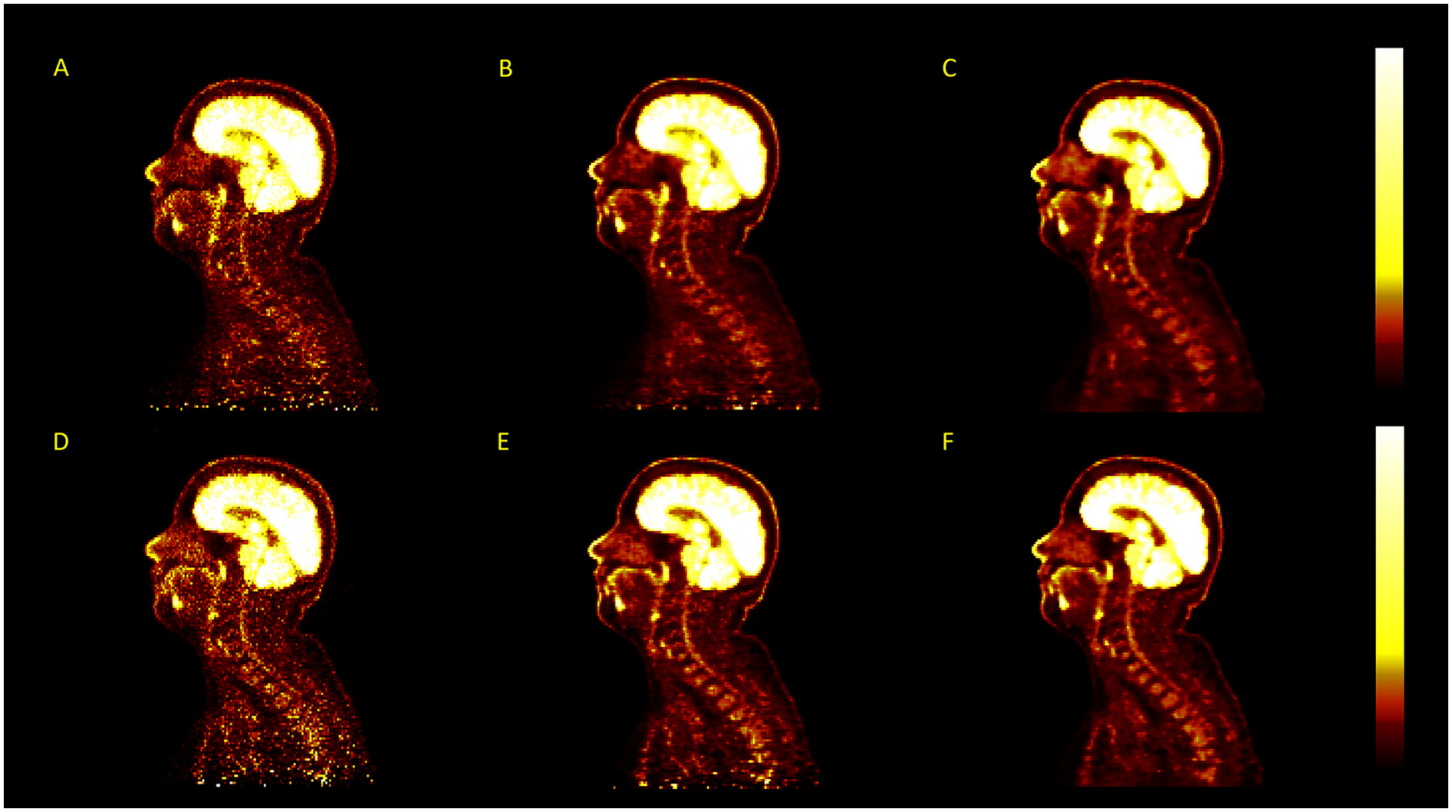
Examples of PET images reconstructed using different image reconstruction algorithms for the same acquisition, with no post-filtering applied, displayed in ${\mathrm{SUV}}_{\mathrm{bw}}$. The algorithms used are OSEM (A), OSEM-PSF (B), Q.Clear (C), TOFOSEM (D), TOFOSEM-PSF (E), and TOF Q.Clear (F).
